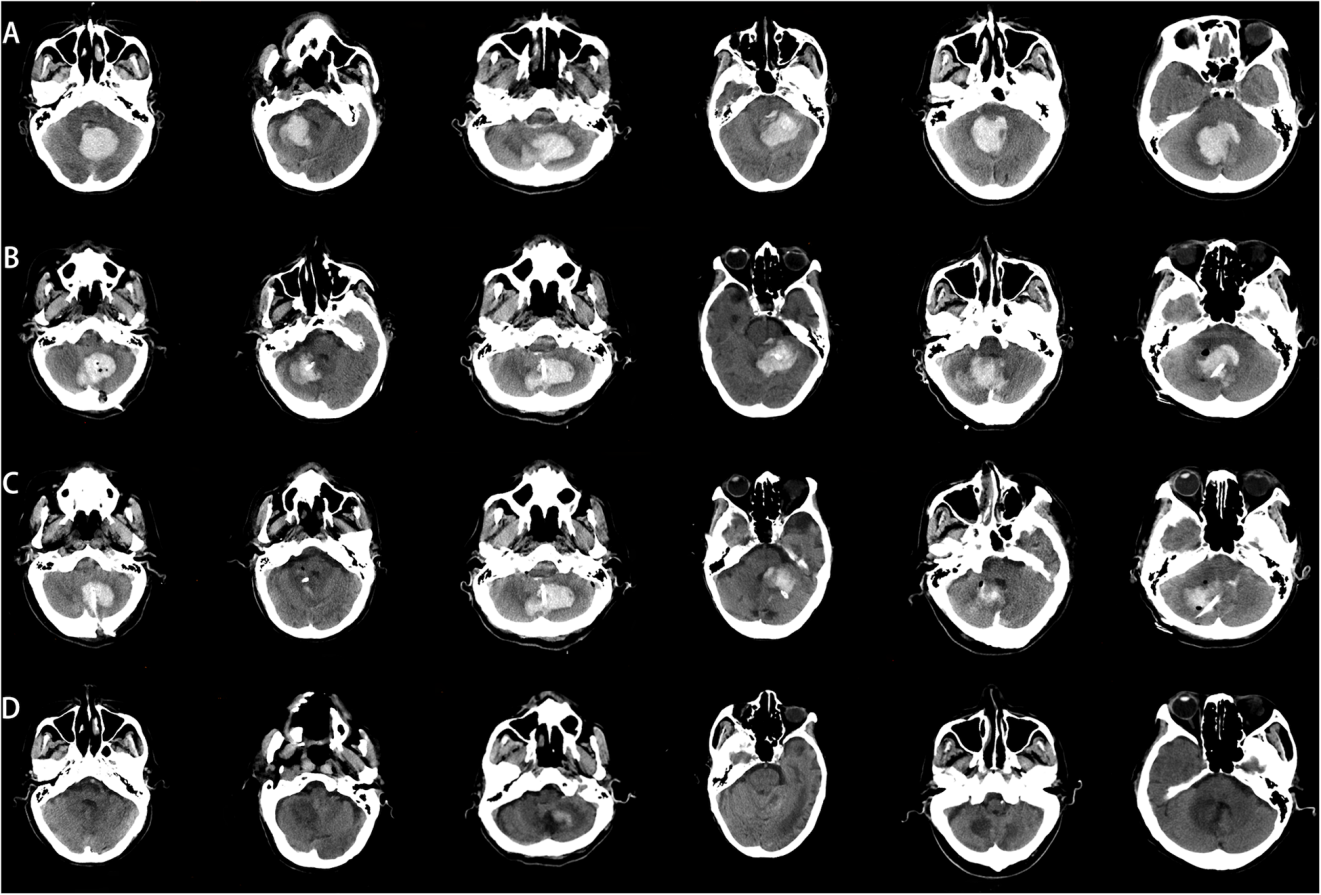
Comparison of preoperative and postoperative CT images. (A) Preoperative CT image, (B) CT image 24 h postoperatively, (C) CT image 3 days postoperatively. (D) CT image 15 days postoperatively.
